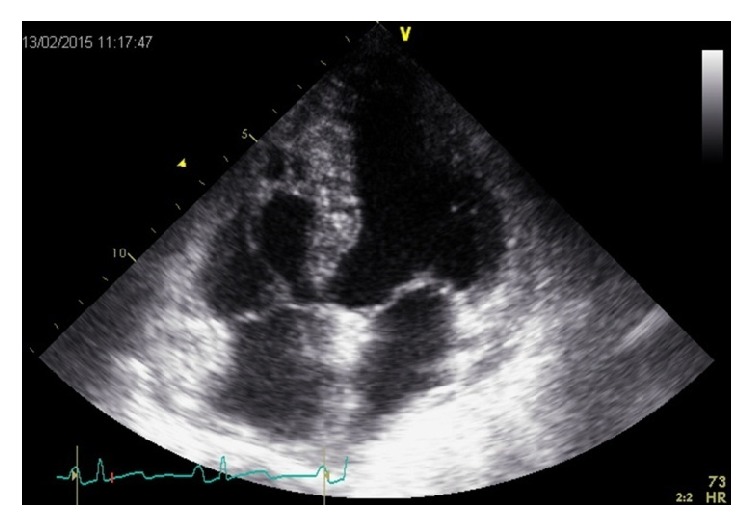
Transthoracic echocardiography apical four-chamber view at discharge with no evidence of intracardiac thrombus or right ventricle dysfunction.
